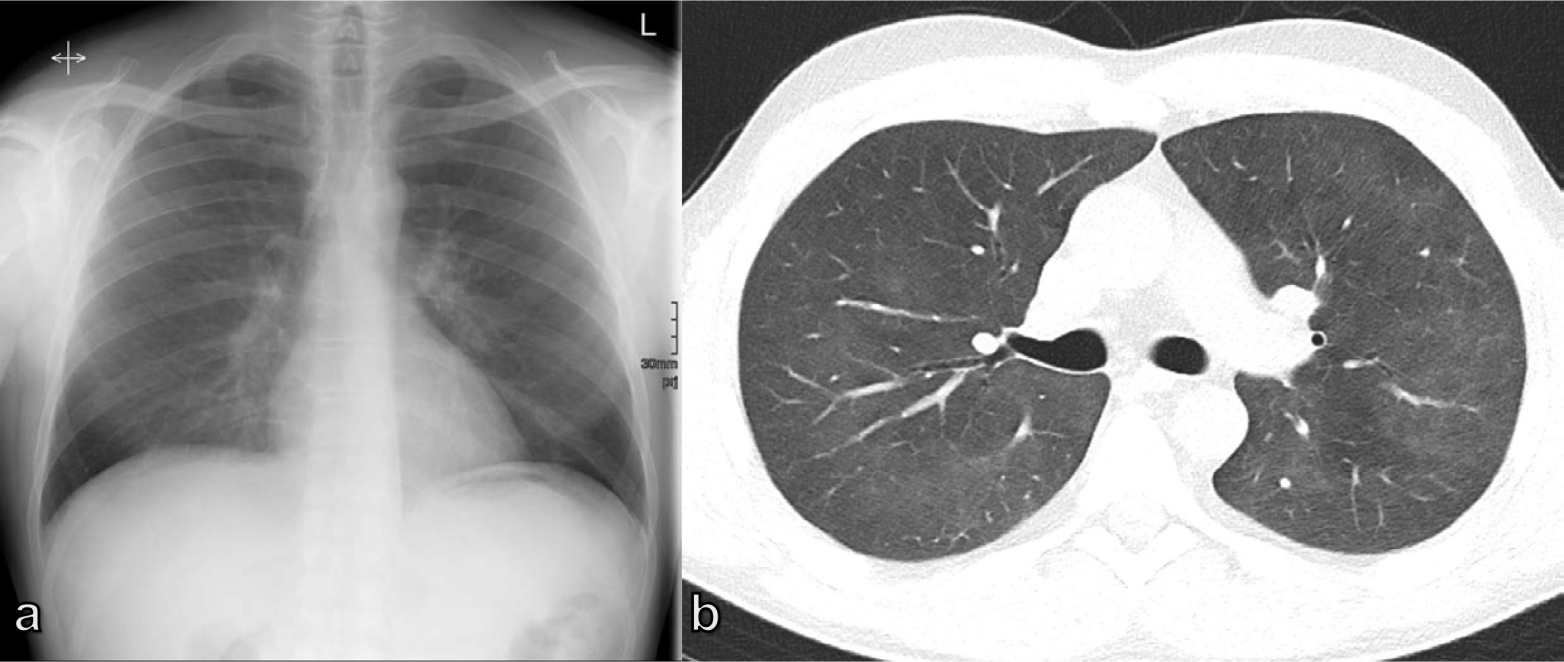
40-year-old male dyspneic patient after kidney transplant and with B-cell lymphoma under chemotherapy and an unremarkable CXR. On CT, diffuse ground-glass opacities as a radiological manifestation of Pneumocystis jirovecii pneumonia. CXR, chest X-ray.
